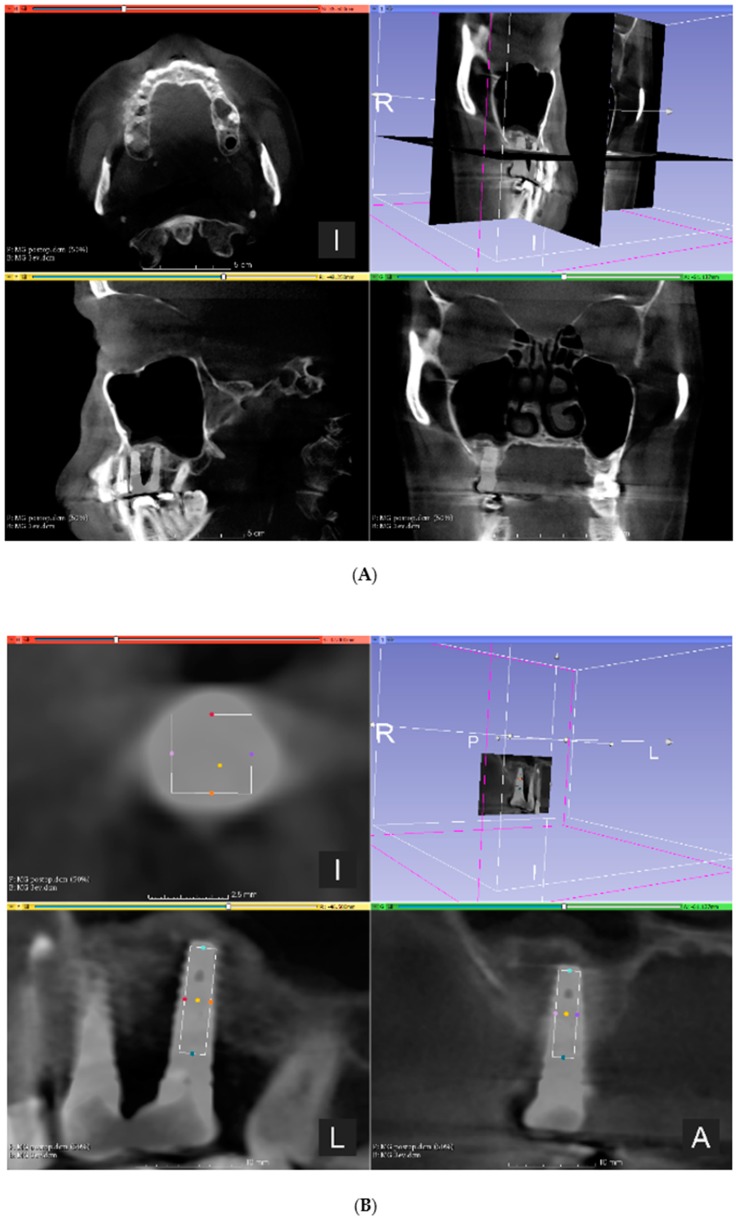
(A) The fused image of the postoperative and 3-year control cone-beam computed tomography (CBCT); (B) Identification of the region of interest (ROI) (position of the biopsy sample harvested during re-entry prior to dental implant placement) on the fused image is presented.
